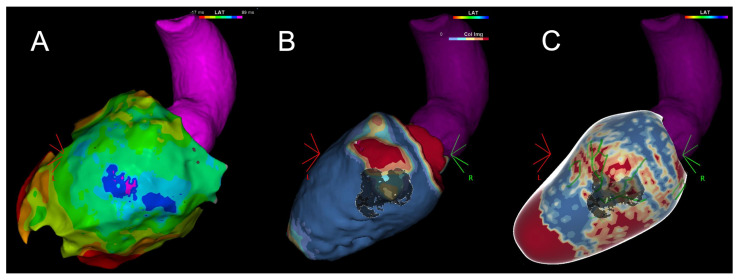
(A) Epicardial isochronal LAT map showing delayed potentials at the mid-basal inferior/inferolateral wall. (B) LVWT MDCT showing thinned areas and the superimposed lipomatous metaplasia. (C) LGE-CMR processed with ADAS3D showing the epicardial scar and border zone areas with heterogeneous tissue channels (green). LAT: local activation mapping, LVWT: left ventricle wall thickness, MDCT: multi-detector computed tomography, LGE-CMR: late gadolinium enhancement cardiac magnetic resonance.
